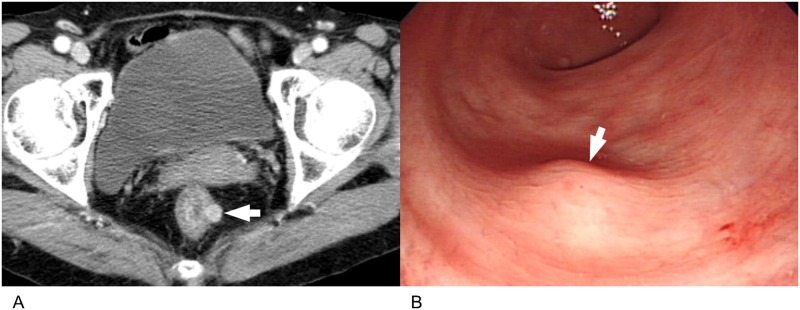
A 76-year-old woman with rectal schwannoma. (A). On portal phase axial CT image, a 1.1 cm round nodule (arrow) is seen at the rectum. The lesion shows homogeneously higher attenuation than back muscle with smooth margin. (B). Colonoscopy shows a smooth elevated subepithelial lesion (arrow) in the rectum.
